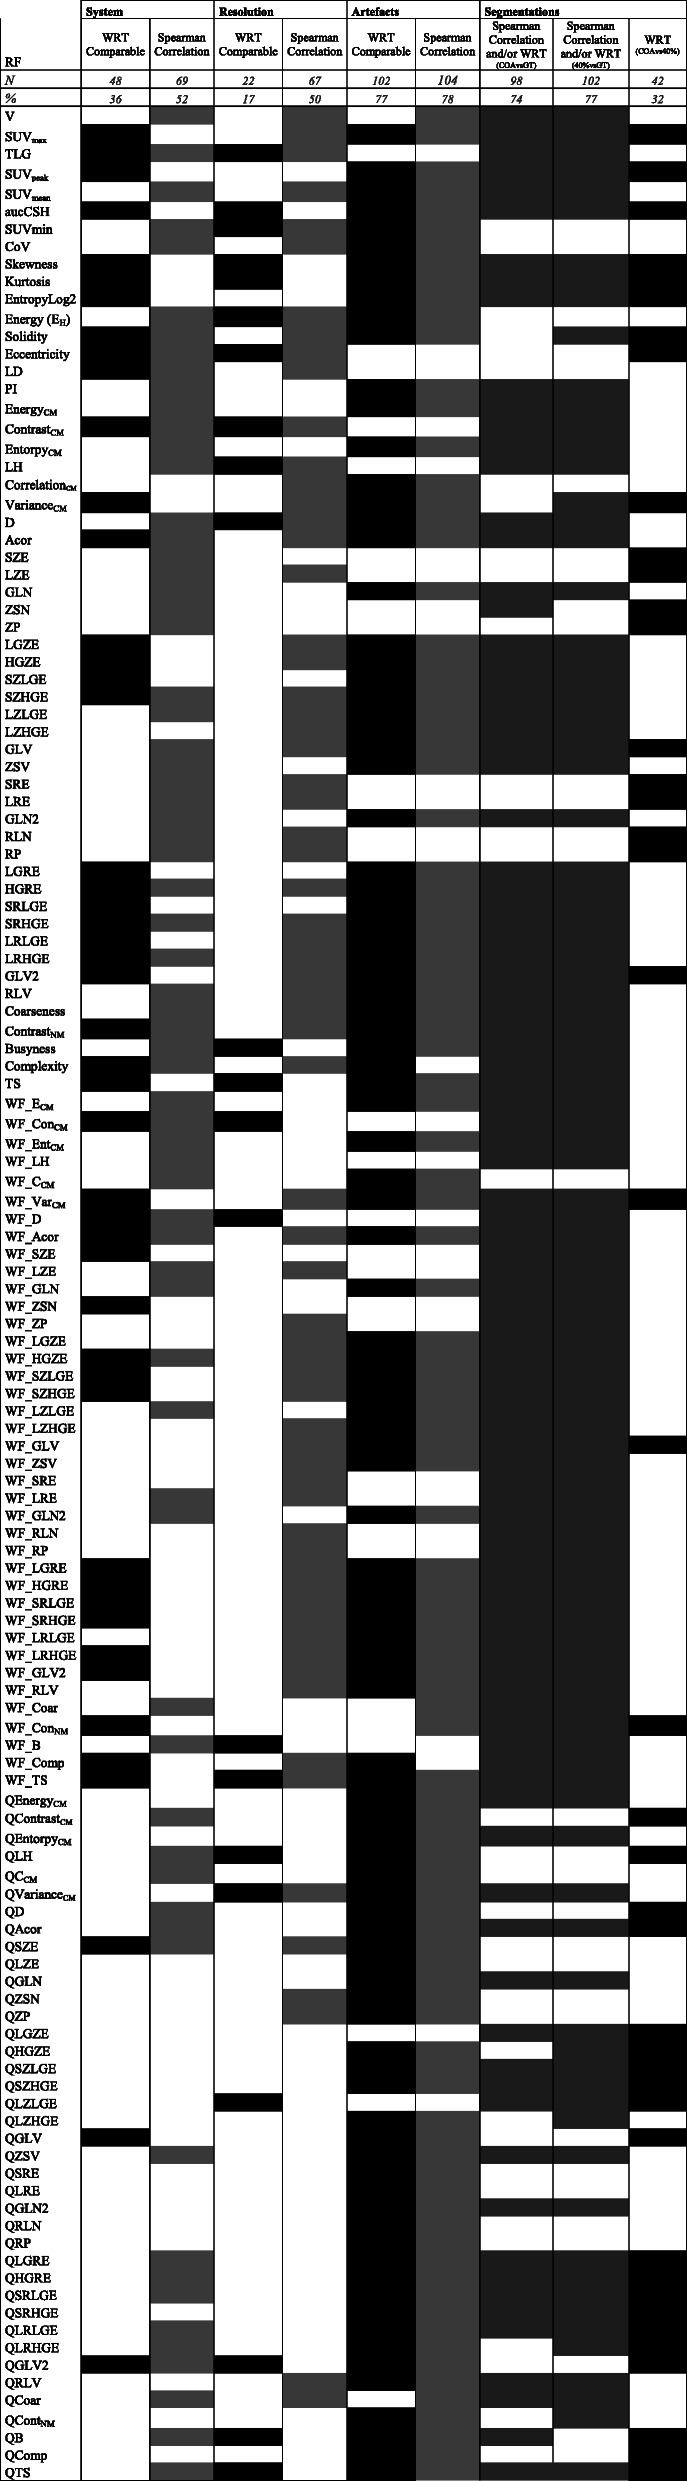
Results of RF analysis. Filled box means positive result for the analysis described on the first row (black is comparable, and gray is strongly correlated) and represents the property of interest, like for example, RF robust to the different PET/CT systems (second column)
